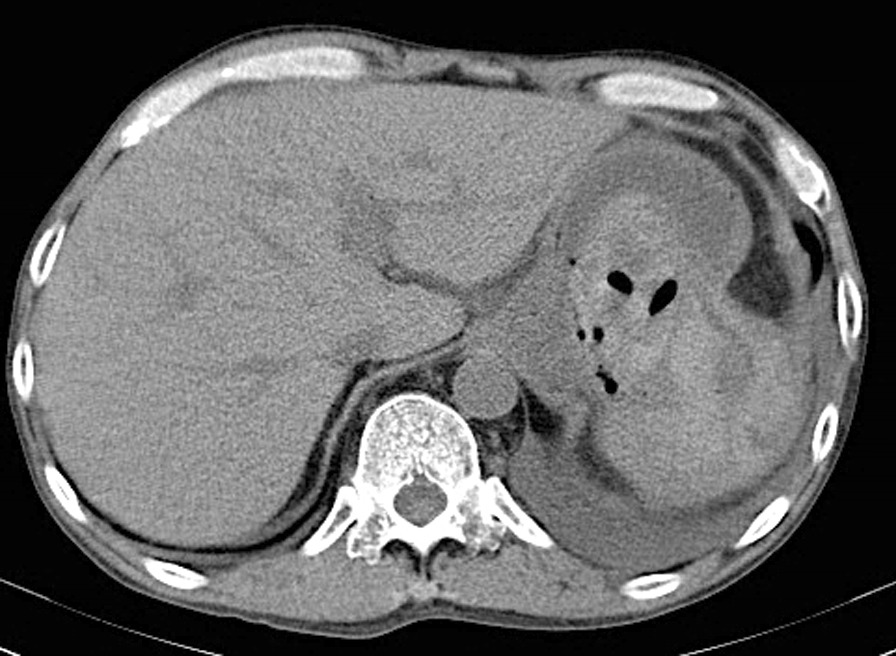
Penetration of pancreatic cyst into the stomach on CT. On CT, a clot in the stomach and a fistula to a pancreatic cyst were identified
